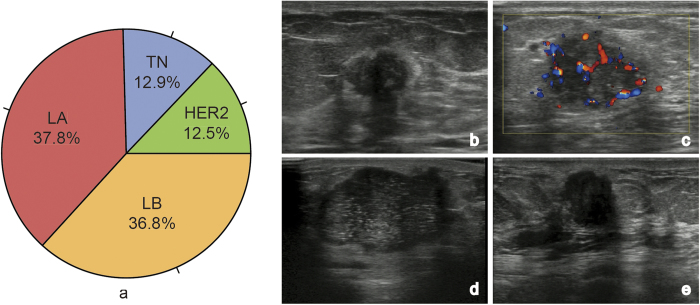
a : A pie chart of the four breast cancer subtypes. b–e are the ultrasound pictures of the LA, LB, HER2 and TN subtypes, respectively. b An LA patient showing the halo and post-acoustic shadowing. c An LB patient with vascularity. d An HER2 patient with calcification and enhanced post-acoustic. e A TN patient showing the micro-lobulate margin and oval shape.
